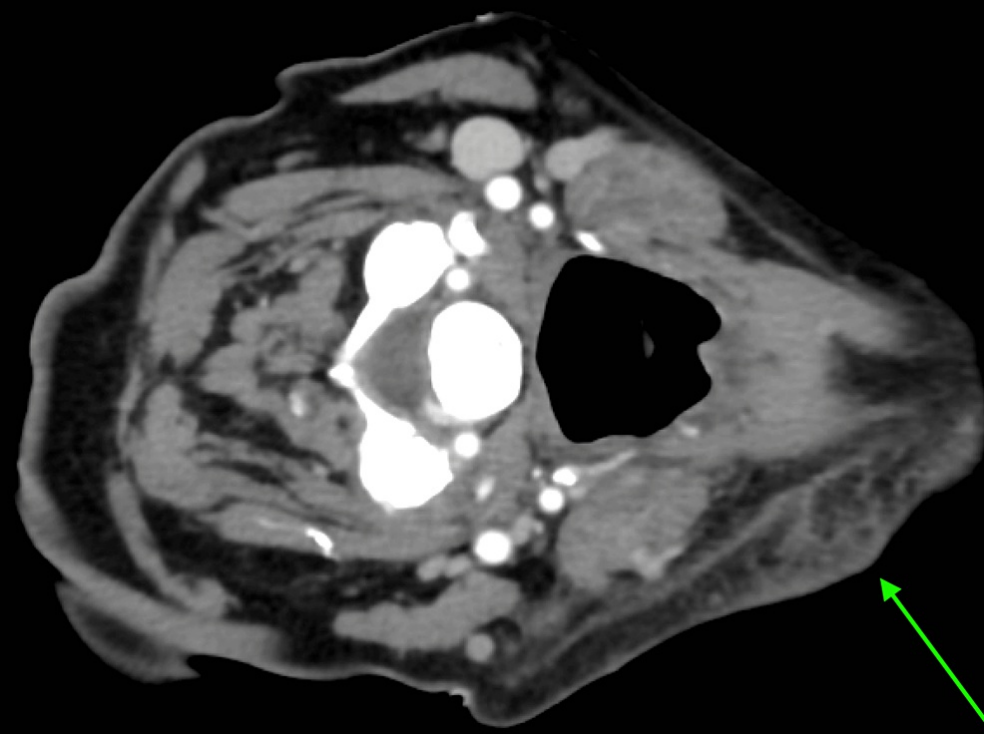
Neck CT, transverse cut, showing cellulitis (rotated 90 degrees clockwise). CT: computed tomography
